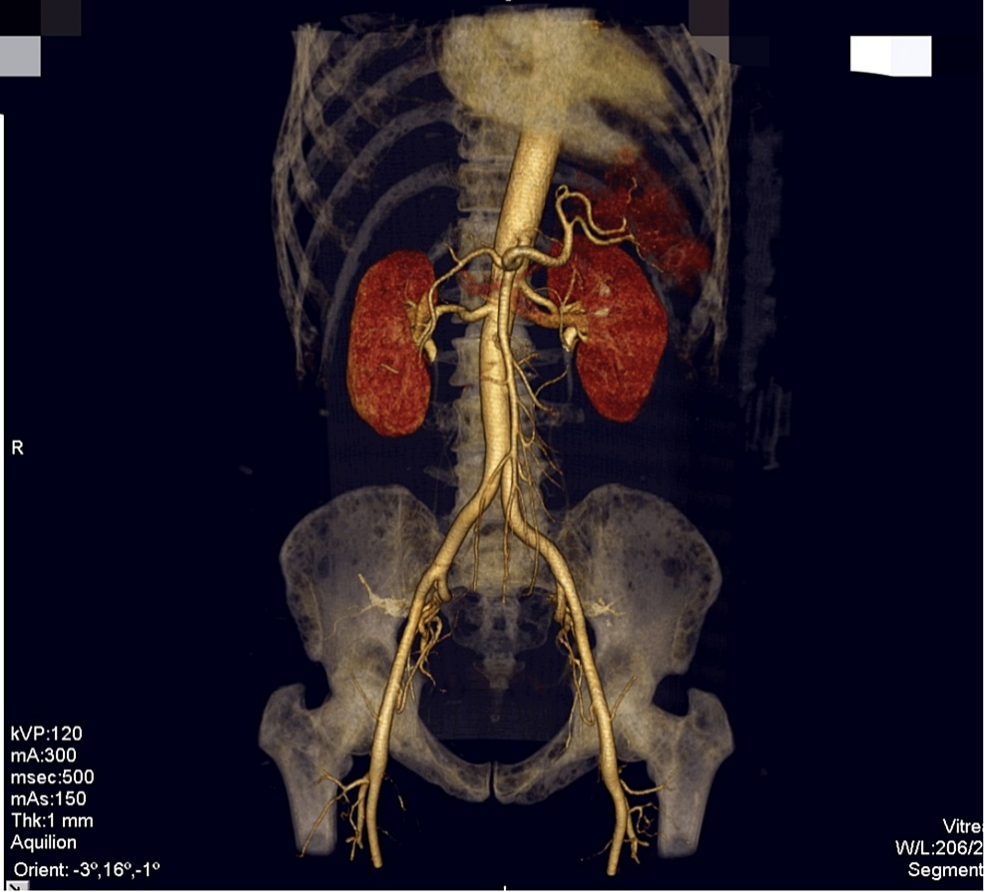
Computed tomography angiography of the abdomen indicating lytic lesions in the bone.
